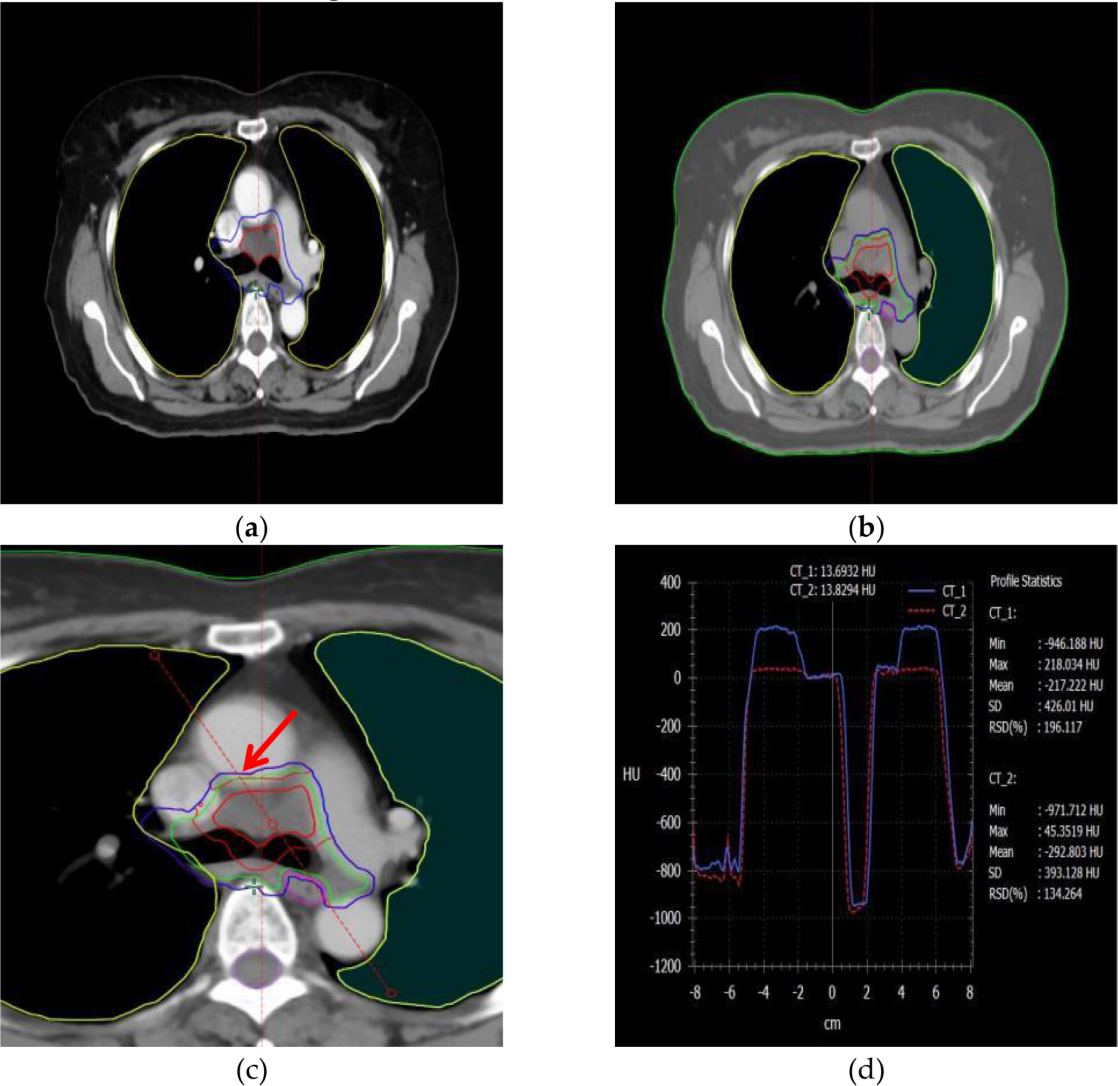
The information on the PTV and OAR structures, as well as the HU distribution curves around the target area, in plain and contrast-enhanced CT modalities. (a) PTV structure in the contrast-enhanced imaging modality, (b) PTV structure in the non-contrast (plain scan) imaging modality, (c) A reference line is drawn through the PTV and organs at risk (OARs) to analyze HU variations, (d) Changes in Hounsfield Units (HU) along the reference line under different imaging modalities.
